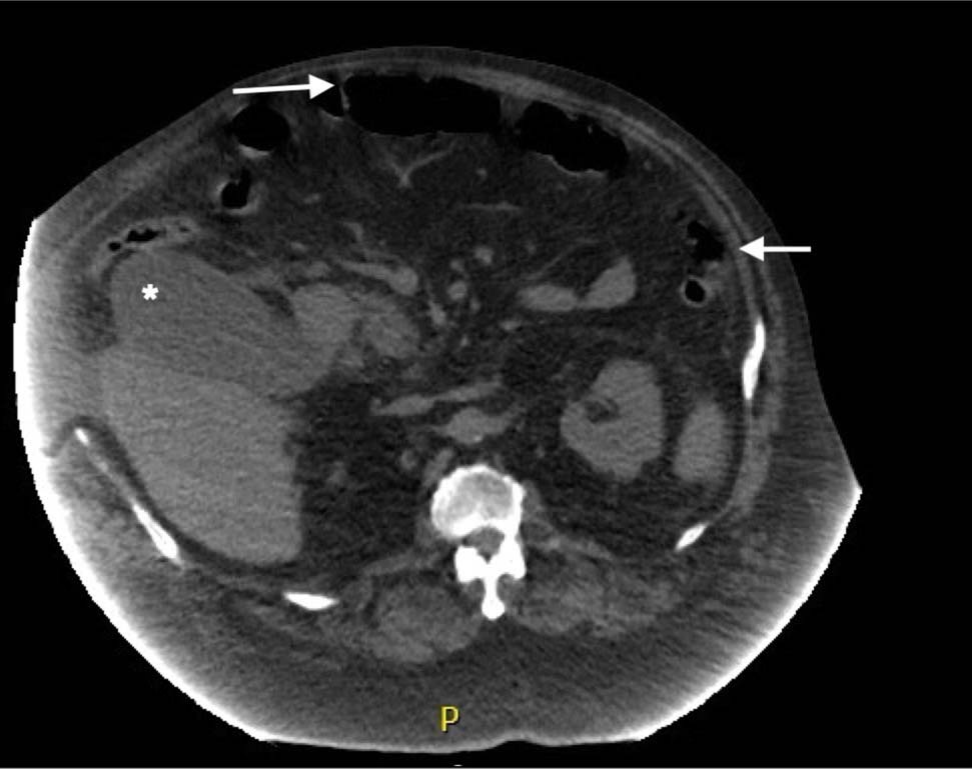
Abdominal computed tomography scan. The axial section reveals the presence of free intraperitoneal air, accompanied by the notable presentation of a distended and edematous gallbladder. Arrows pointing toward free intraperitoneal air. Asterisk showing distended and edematous gallbladder.
